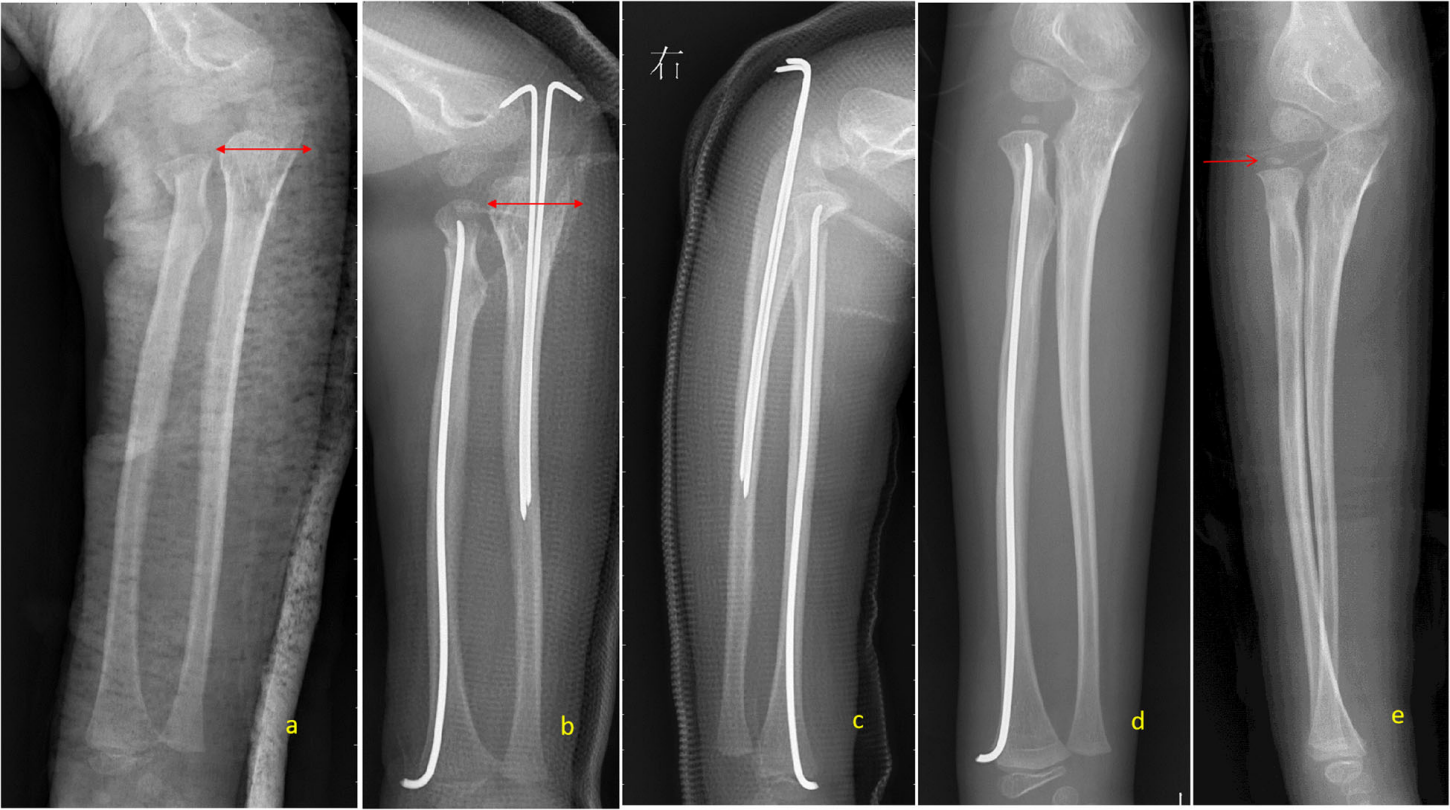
A 7-year-old boy classified type IV injury in right elbow. a A-P view of X-ray film show fracture in coronal plane of olecranon with displacement less than one third of ulna (arrow) associated with radial neck fracture with angulation less than 60°. b, c A-P and lateral view of X-ray film show this boy was treated with close reduction and internal fixation with K-wire for olecranon and close reduction and internal fixation with ESIN for radial neck, but the broad of olecranon in coronal plane was not corrected (arrow). d Three months postoperation, A-P view of X-ray film show the radial neck fracture healed but malunion of olecranon. e Six months postoperation, A-P view of X-ray film show radial head subluxation could be observed after the ESIN removed because of the angulated malunion of olecranon when pronate, only pronation of right forearm was limited
